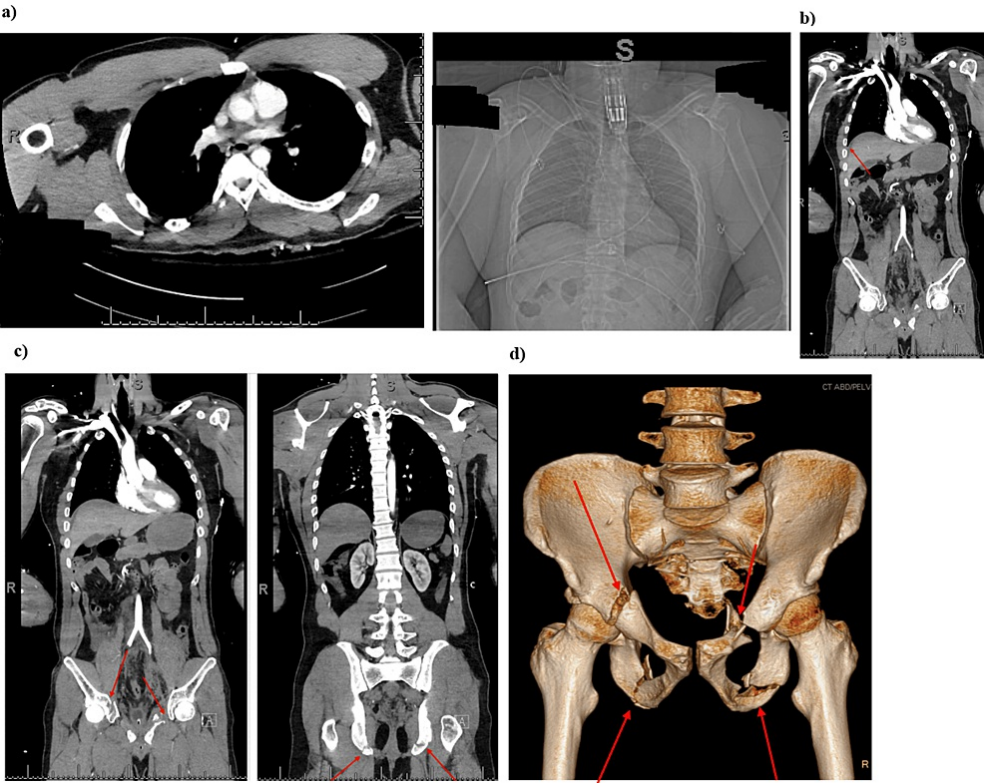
CT scans of the chest, abdomen, and pelvis. Axial and coronal CT images of the thorax, abdomen, and pelvis were obtained after the uneventful administration of IV contrast. One hundred milliliters of Isovue 370 was injected. (a) No cardiomegaly or pericardial effusion present. There is no mediastinal lymphadenopathy. There is no evidence of aortic dissection or thoracic aortic aneurysm. There are no hilar or adnexal masses. There is no focal airspace consolidation, pleural effusion, or pneumothorax. There is no acute displaced rib fracture. Sternum is intact. Thoracic vertebrae appear intact. (b) Free fluid noted within the pelvis and presacral space and the paracolic gutters. Hyperdense fluid noted within the extraperitoneal pelvis and intraperitoneal locations. The abdominal aorta is nonaneurysmal. Free air is noted within the abdomen. Underlying perforation to hollow viscus given consideration (indicated by red arrow). The presence of free fluid in the pelvis and intraperitoneal space on CT imaging raised concern for possible associated bladder or urethral injuries, necessitating ongoing monitoring and potential intervention. (c) There are extensive pelvic fractures. Fractures are noted involving the left superior and inferior pubic rami. Fractures are noted involving the right acetabulum and right inferior pubic ramus. There is also a fracture involving the right superior pubic ramus. No femoral head dislocation. No osseous fractures noted involving the sacrum posteriorly. Blood products found within the intraperitoneal and extraperitoneal pelvic space. Points of fracture are indicated with red arrows on CT. (d) 3D rendering isolating the skeletal abnormalities. Sites of fractures are indicated by red arrows.
